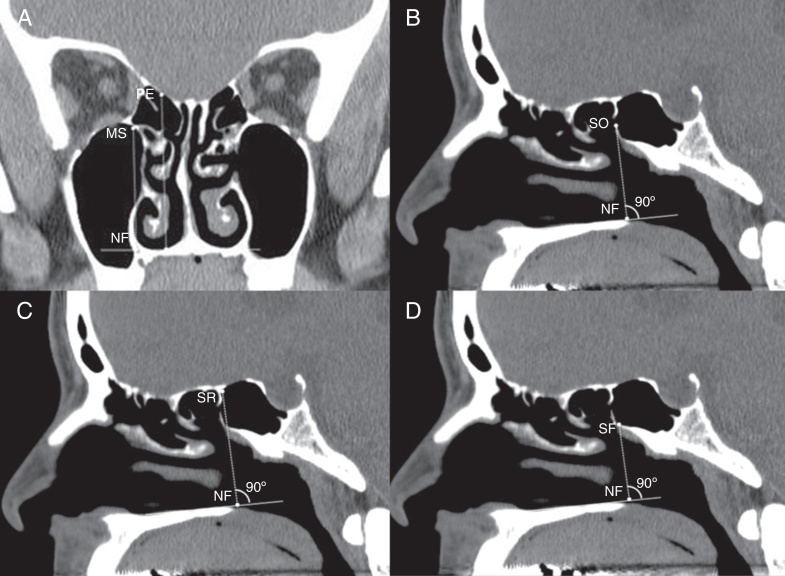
(A) Coronal CT image demonstrating the vertical distance from the nasal floor (NF) to the highest medial maxillary sinus roof (MS) and posterior ethmoid skull base (PE). (B) Sagittal CT image demonstrating the vertical distance from the nasal floor (NF) to the sphenoid ostium (SO). (C) Sagittal CT image demonstrating the vertical distance from the nasal floor (NF) to the anterior sphenoid sinus roof (SR). (D) Sagittal CT image demonstrating the vertical distance from the nasal floor (NF) to the anterior sphenoid sinus floor (SF).2.Vertical distance from the inferior margin of the sphenoid ostium to the hMMSR (SO-MS). Bony opening at the sphenoid face was identified both on axial and sagittal CT images. The sagittal slice centered on the sphenoid ostium was used to measure the vertical distance from the nasal floor to the sphenoid ostium (SO-NF) (Fig. 1B). SO-MS was calculated by subtracting MS-NF from SO-NF. A sphenoid ostium inferior to the hMMSR was given a negative value.3.Vertical distance from the anterior sphenoid sinus roof to the hMMSR (SR-MS). The sinus roof was identified on a same sagittal CT slice centered on the sphenoid ostium. The vertical distance from the nasal floor to sphenoid sinus roof was measured (SR-NF) (Fig. 1C). If an Onodi cell was found, the vertical distance was measured from the nasal floor to the skull base at the coronal plane of the anterior sphenoid face. SR-MS was calculated by subtracting MS-NF from SR-NF.4.Vertical distance from the hMMSR to the anterior sphenoid sinus floor (MS-SF). The sinus floor was identified on a same sagittal CT slice centered on the sphenoid ostium. The vertical distance from the nasal floor to sphenoid sinus floor was measured (SF-NF) (Fig. 1D). MS-SF was calculated by subtracting SF-NF from MS-NF.5.The coronal distance from the posterior wall of the maxillary sinus to the sphenoid ostium. Triplanar display of CT data in axial, sagittal, and coronal planes was simultaneously viewed and analyzed. The sphenoid ostium in the axial plane was identified. The most posterior point of the posterior wall of the maxillary sinus was identified by scrolling through the sagittal images. It was usually located at the medial part of maxillary sinus. Using the Picture Archiving and Communication System (PACS) cross-referencing tool, the relationship between the most posterior point of the posterior wall of the maxillary sinus in the sagittal plane and the sphenoid ostium in the axial plane was determined. The sphenoid ostium was behind (Fig. 2A) or in fronts of (Fig. 2B) the coronal plane of the most posterior point of the posterior wall of the maxillary sinus. Or it was in the same plane (Fig. 2C). The distance between the two structures was determined by the number of the intervening 2 mm-thick coronal slices between them. A sphenoid ostium anterior to the coronal plane of the most posterior point of the posterior wall of the maxillary sinus was given a negative value.
